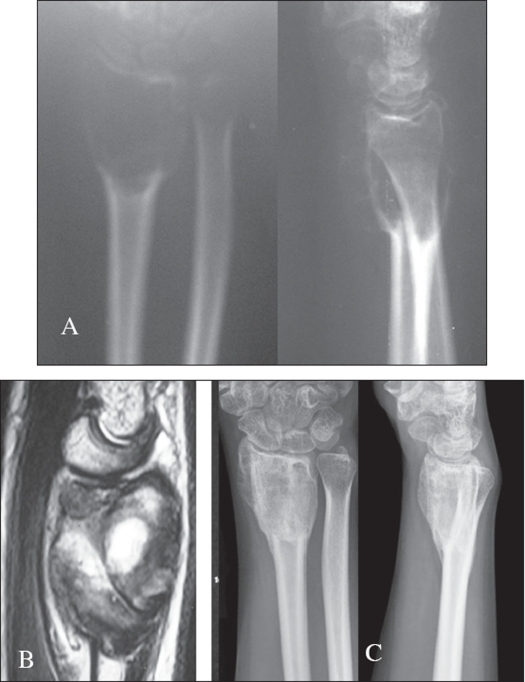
A) X-ray (A.P. and lateral), B) MRI of GCT of lower end radius, C) Two years followup X-ray of the same after intra lesional curettage and bone grafting showing healed lesion.
